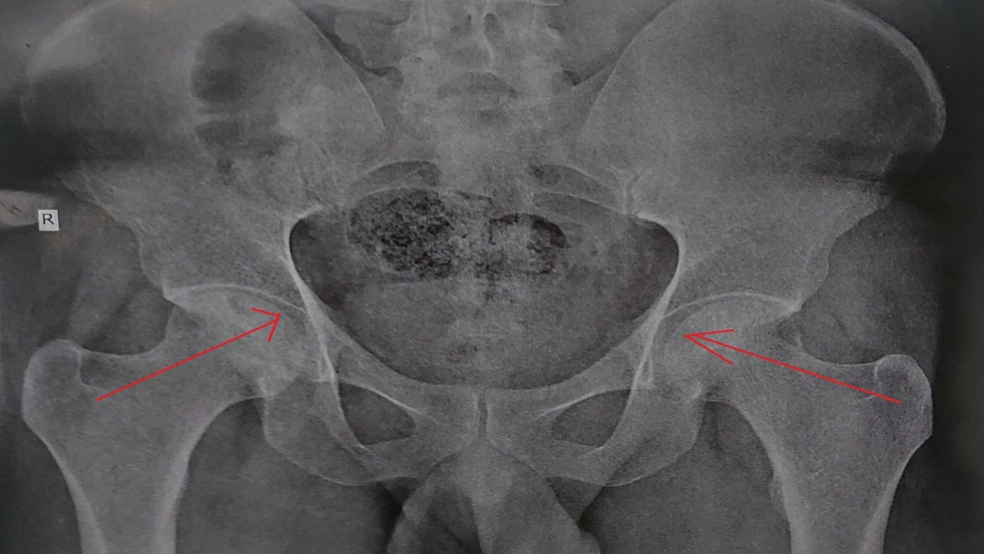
X-ray of a 28-year-old male in the anterior-posterior view shows the avascular necrosis of the bilateral head of the femur
